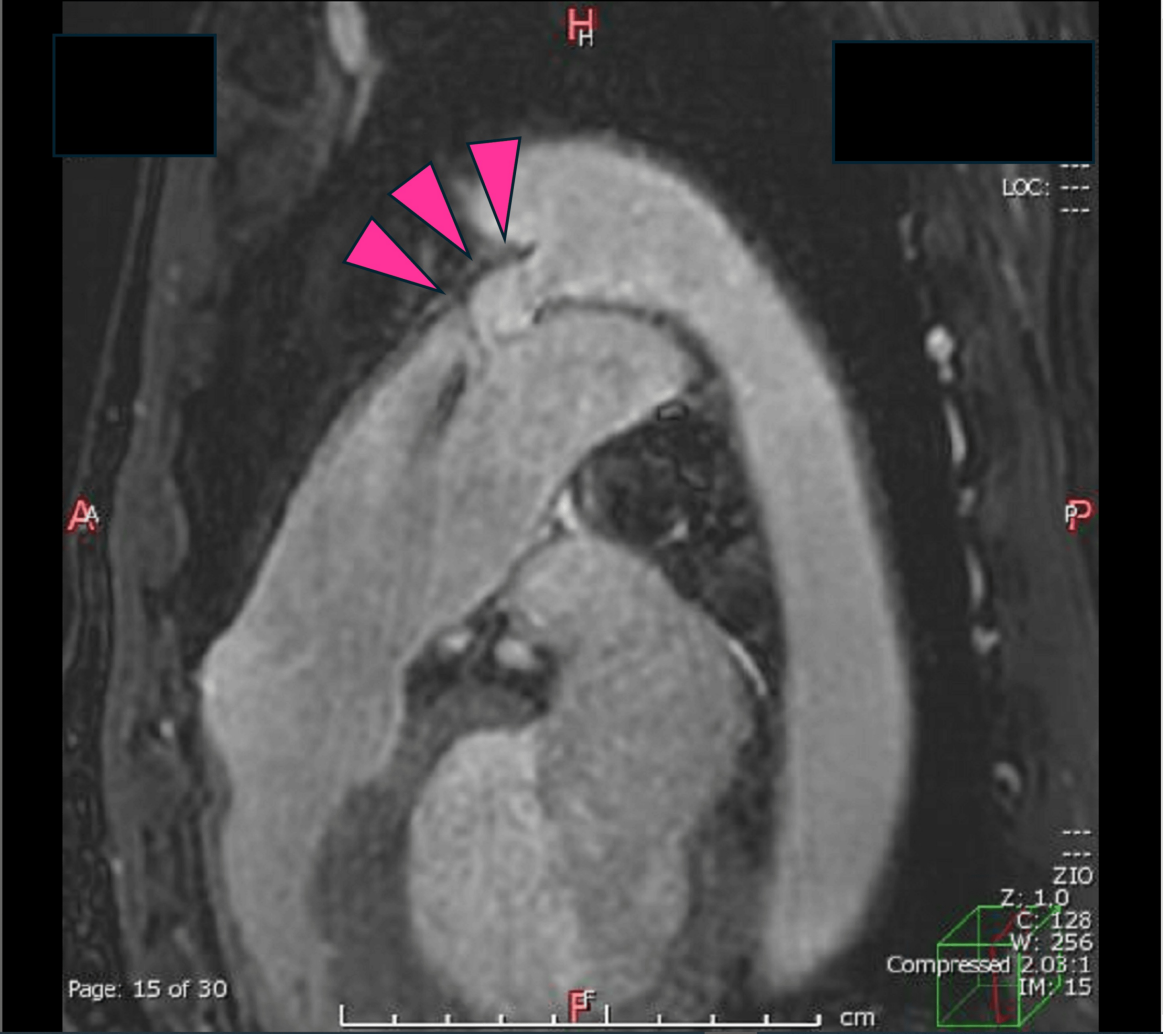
Magnetic resonance imaging of the aorta revealed an aneurysmal patent ductus arteriosus (Krichenko classification, type D).
